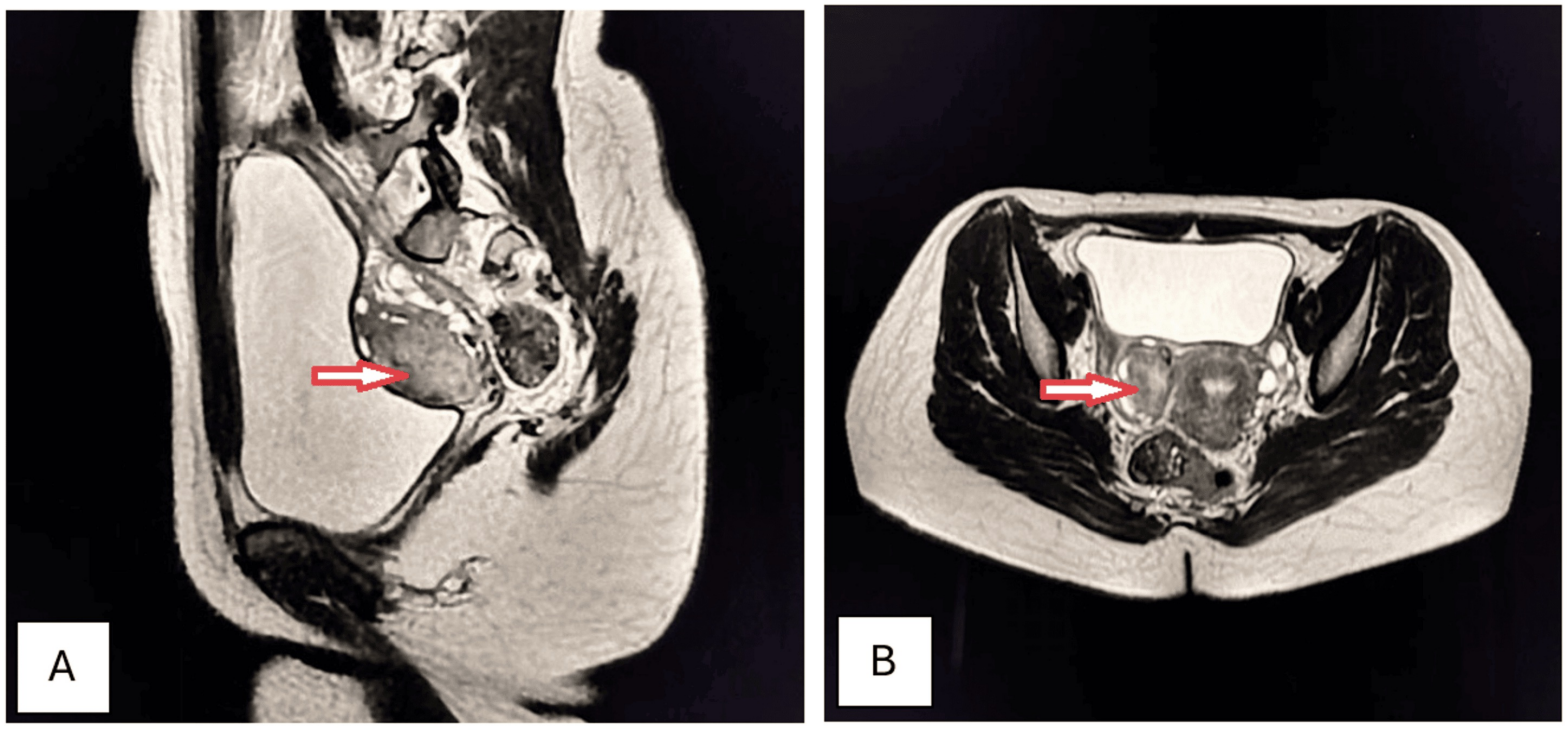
Fibroma of the right ovary (arrows). Sagittal (A) and axial (B) T2W MRI imaging shows a well-defined, round-to-oval, solid mass with a thick hypointense rim (black garland sign) with relative sparing of the central ovarian parenchyma. This iso- to hyperintense mass noted in the right ovary is displacing ovarian follicles peripherally, which is consistent with a fibroma. T2W: T2-weighted
